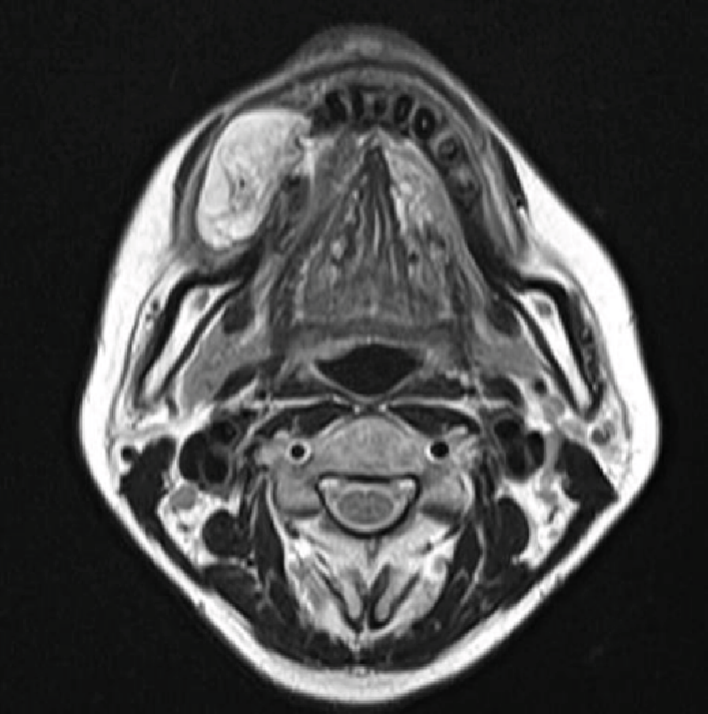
T2-weighted magnetic resonance image. The tumor shows high signal intensity on the T2-weighted image and an oval-shaped neoplastic lesion with a well-defined border of 40 mm × 20 mm.
